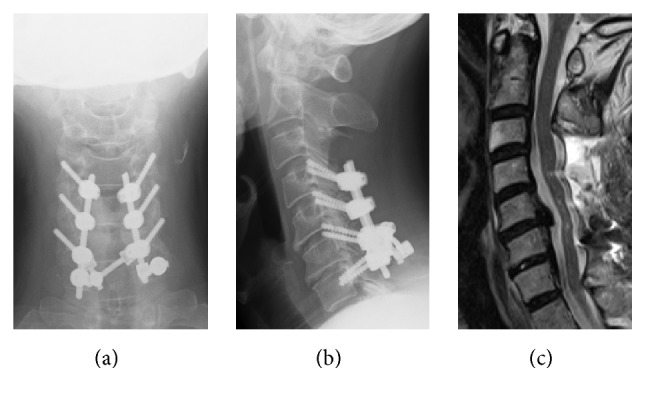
Postoperative X-ray and magnetic resonance imaging. (a) and (b): anteroposterior and lateral view X-ray of the cervical spine. (c): magnetic resonance imaging showed sufficient decompression of the spinal cord and posterior shifting, without high-intensity areas at C3/4.
